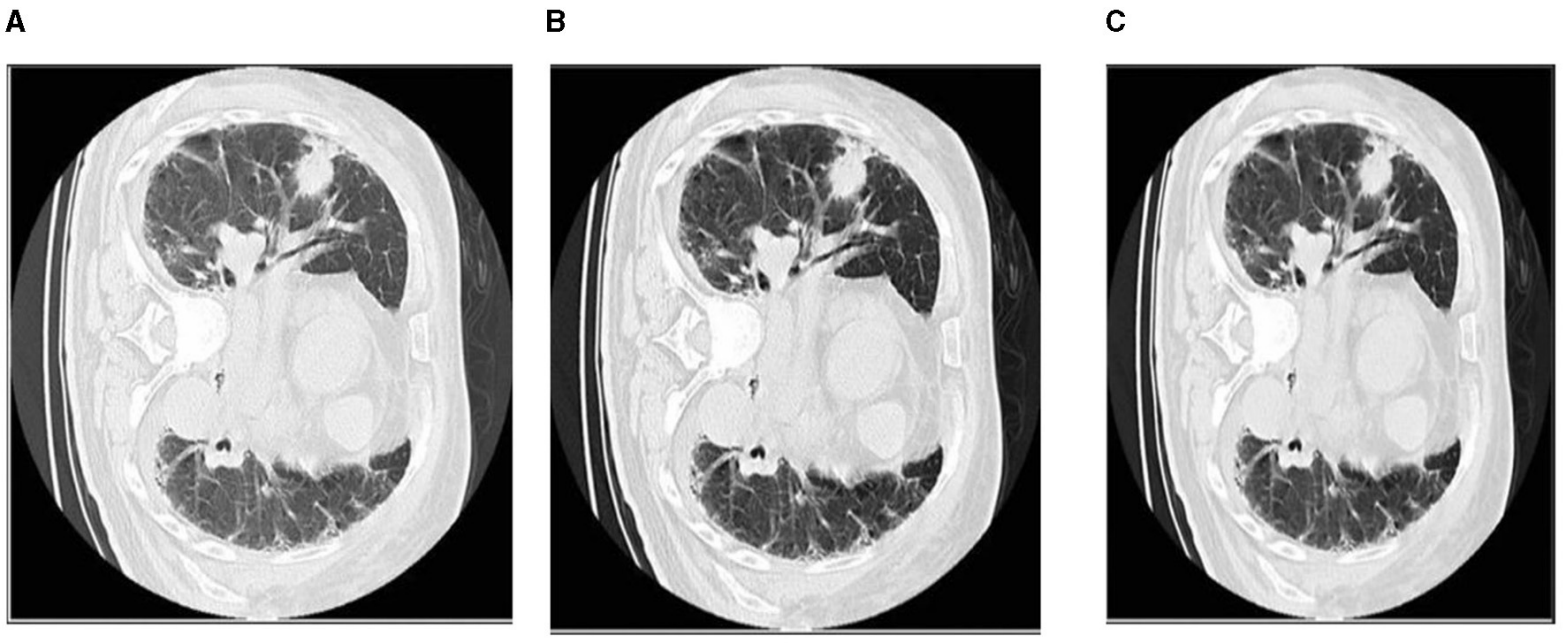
The first picture from left to right shows how the Wiener filter works with CLAHE. (A) Original CT scan image. (B) CT image with CLAHE image. (C) CLAHE with Weiner filter.
